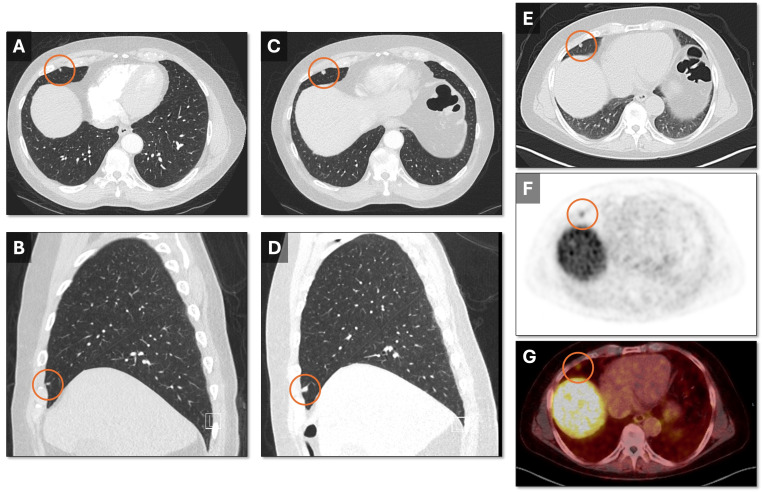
Empirically treated right middle lobe costal pleural nodule in a patient with a history of prostate cancer. CT images at baseline in (A) axial and (B) coronal, 5 years later from baseline in (C) axial and (D) coronal, and 5 years from baseline in axial (E) CT, (F) PSMA PET, and (G) PET/CT overlay. Nodule measures 2 mm at baseline, 5 mm at 5-year follow-up on both high-resolution chest CT (C,D) and PSMA PET/CT (E–G). The red circle highlights the concerning pulmonary nodule. Nodule was suspicious for malignancy based on interval growth and PSMA-avidity.
